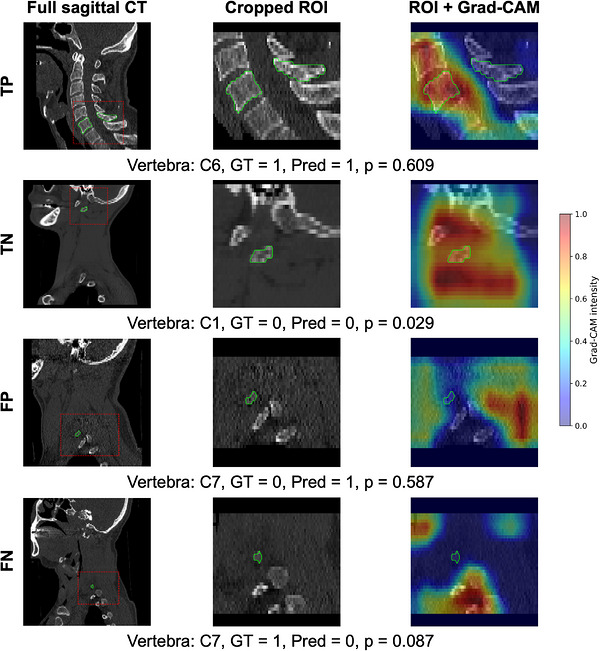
Representative true‐positive (TP), true‐negative (TN), false‐positive (FP), and false‐negative (FN) cases for vertebra‐level fracture classification with Grad‐CAM visualization. Each row shows one representative case. The first column shows the full sagittal CT image, with the source region of the cropped ROI explicitly marked by a red dashed box. The second column shows the cropped ROI, and the third column shows the ROI with Grad‐CAM overlaid. Detailed case information, including vertebral level, ground‐truth label, predicted label, and predicted fracture probability, is provided below each row. All sagittal panels were regenerated using display‐only resampling to 1.0 × 1.0 mm in‐plane resolution to ensure square physical pixels.
